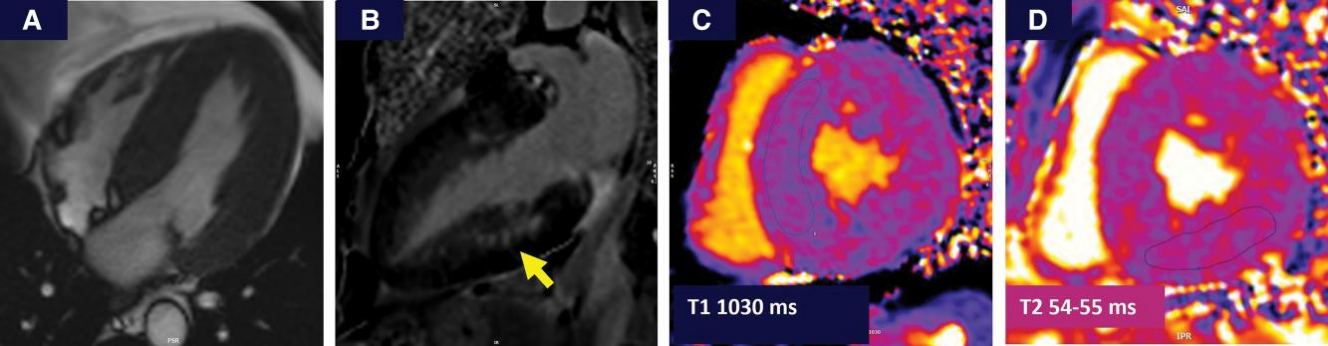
Cardiac magnetic resonance aspects. (*A*) Apical four-chamber view showing concentric severe left ventricular hypertrophy with a maximum wall thickness of 22 mm; (*B*) mid-myocardial late gadolinium enhancement in the basal and mid segments of inferior walls; (*C*) normal native T1 values (1030 ms); (*D*) normal global T2 values (46 ms) and elevated values in late gadolinium enhancement areas (54–55 ms).
